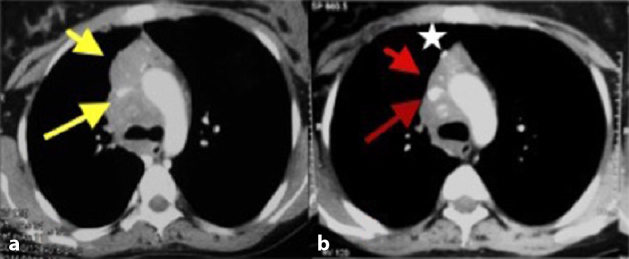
Pre-treatment (a) and post-treatment (1 year; b) axial post-contrast CT images showing significant interval regression of the mass (short arrows in a and b) and superior vena cava luminal compromise (long arrows in a and b) following treatment. The post-biopsy metallic clip is noteworthy (star in b).
